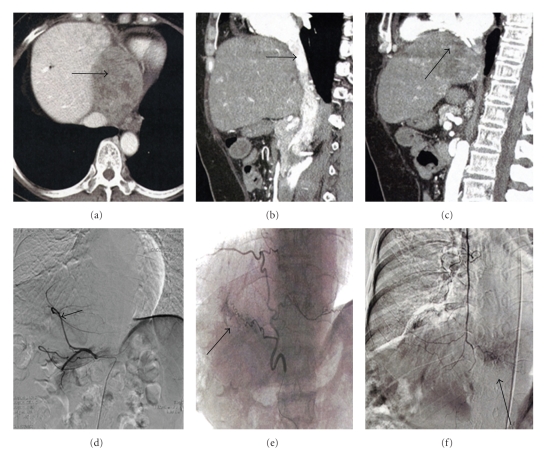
Preoperative findings. CT shows a heterogenous mass ((a)–(c), arrow), 9 cm in diameter adjacent to the remnant liver (a), displacing the IVC (b) and the pericardium (c). Angiography shows that the remnant liver is supplied by the left hepatic artery (d). Selective angiograms show a hypervascular tumor supplied from the descending branch of the inferior phrenic artery (IPA) (e) and the phrenic branch of the internal mammary artery (IMA) (f).
